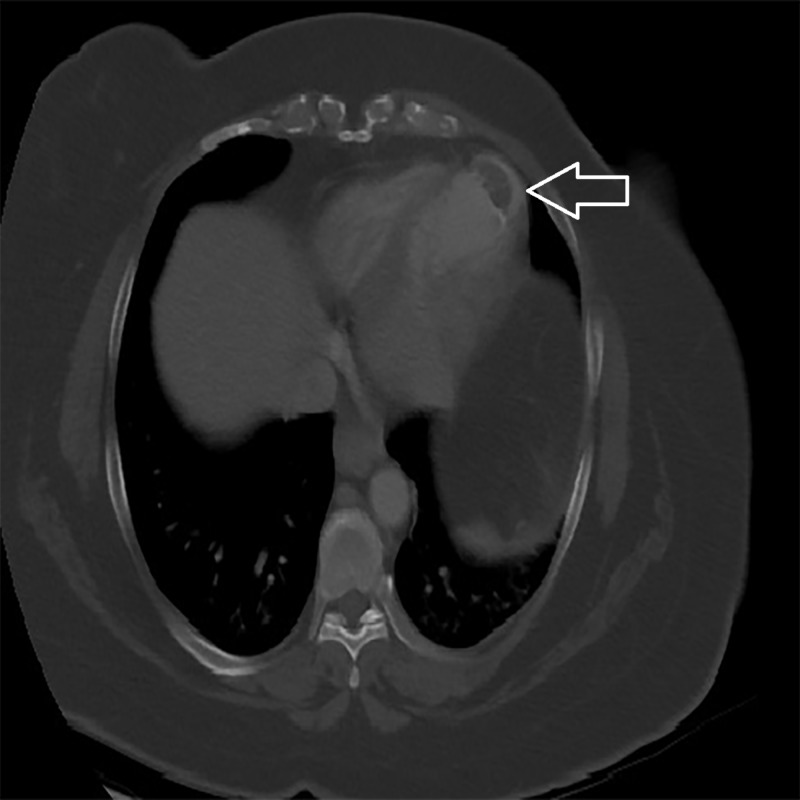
Computed tomography of the abdomen showing mild cardiomegaly, old infarction on the anterior-inferior wall of the left ventricle. There is a 37.47 mm hypodense calcified thrombus along the left ventricular apex (white arrow).
